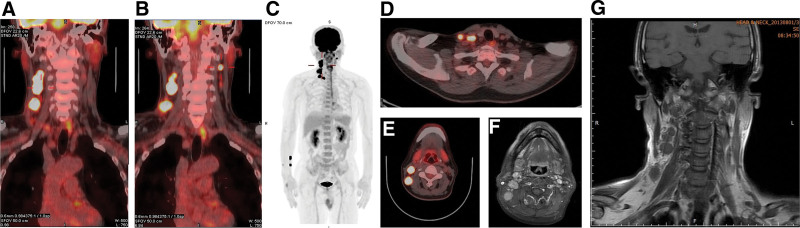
(A–E) The whole-body FDG-PET CT indicated right neck levels II to V enlarged lymphadenopathies and left neck levels II and III normal-sized lymphadenopathies. The FDG-avid pathologic lesions were not found in the gastrointestinal tract, liver, gallbladder, pancreas, adrenal glands, bilateral kidneys, and spleen. (F and G) Head and neck MRI reported right neck enlarged and clustered lymphadenopathy at levels II, III, IV, and Va and supraclavicular. One of the larger lymph nodes at the right inferior jugular chain showed focal intralesional necrotic foci and heterogeneous enhancement. CT = computed tomography, FDG = 2-deoxy-2-[18F]fluoro-D-glucose, MRI = magnetic resonance imaging, PET = positron emission tomography.
